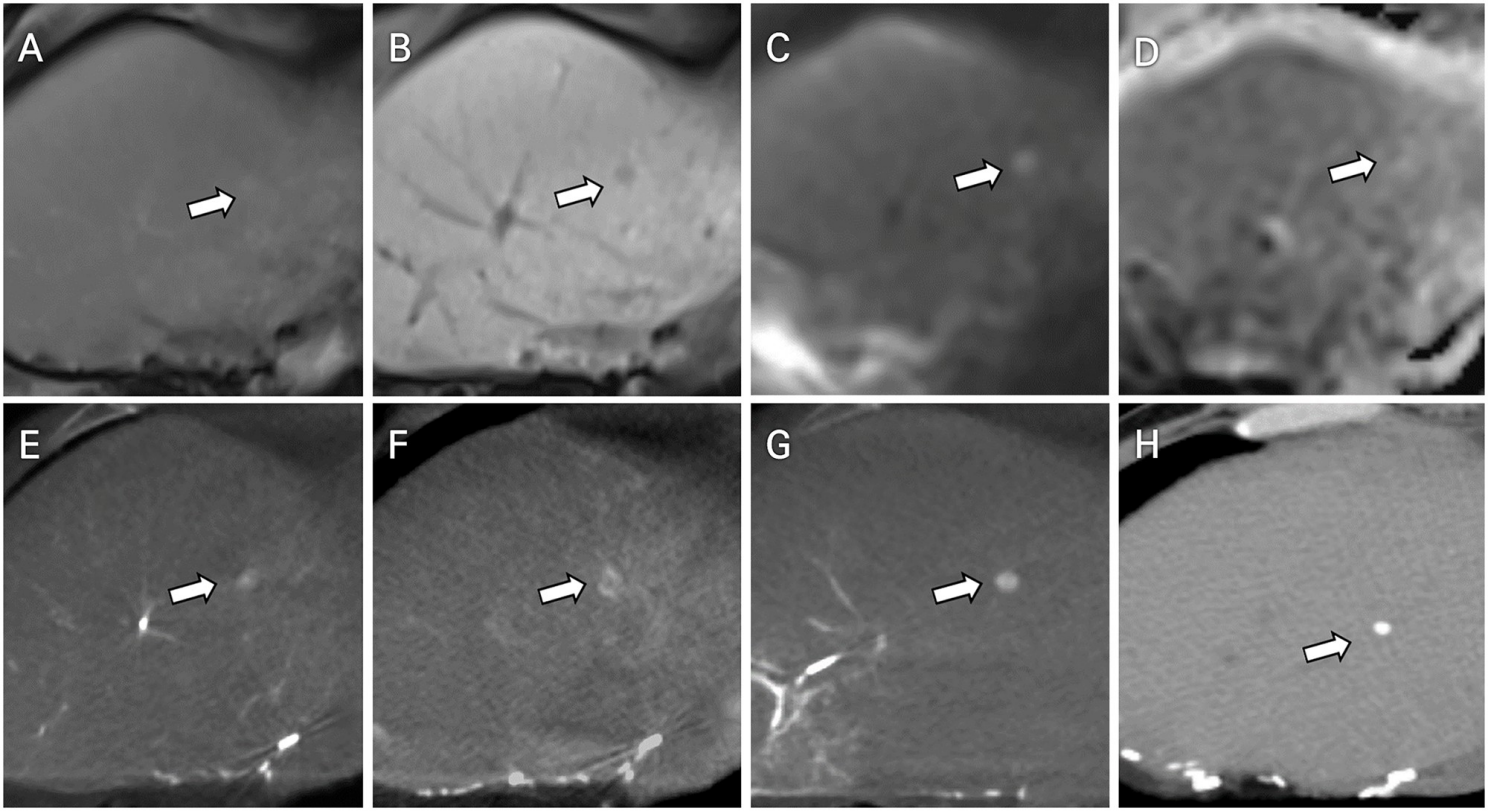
A 53-year-old male patient with right hepatectomy and recurred multifocal HCCs (Child–Pugh class A, BCLC stage B). (A) During contrast-enhanced MRI, a subcentimeter lesion (white arrow) in segment 4 remained nearly unidentified in the AP. (B) This lesion was seen as faint hypointensity on the hepatobiliary phase. (C and D) The tiny correlated lesion (white arrow) showed a high signal on DWI (b = 800) and low value on the ADC map, representing diffusion restriction. (E) AP-CBCT detected a faint hypervascular lesion in segment 4 (white arrow). However, upon interpretation, this lesion was recognized as an arterioportal shunt because of the indistinct margin. (F) This lesion was considered as a treatment target because delayed rim enhancement was noticed on the delayed-phase CBCT image (white arrow). (G) Positive lipiodol uptake was observed on the lipiodol CBCT image when chemoembolization was performed at the subsegmental level (white arrow). (H) Compact lipiodol uptake without residual arterial enhancement was visualized on the dynamic enhanced CT image scanned at the three-month follow-up (white arrow).
